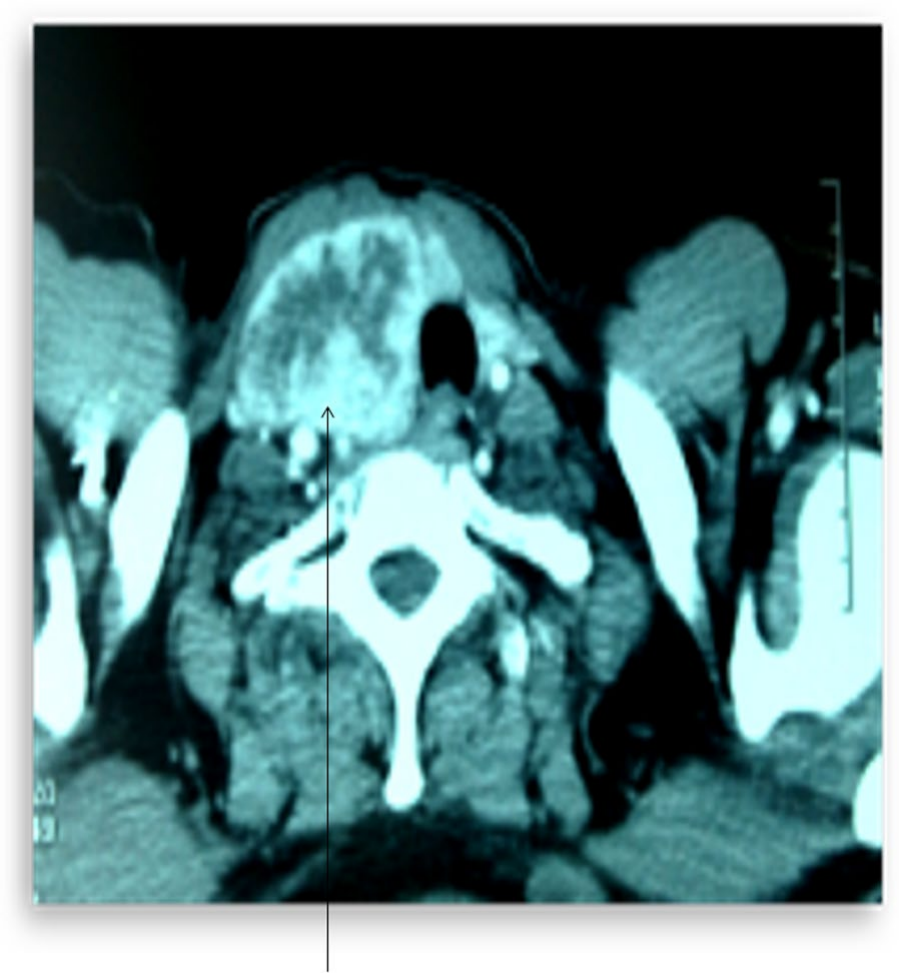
Cervical computed tomography scan—axial cup showing a large nodule in right lobe of thyroid, heterodense, dipping into the upper mediastinum and measuring 8 × 4 cm
